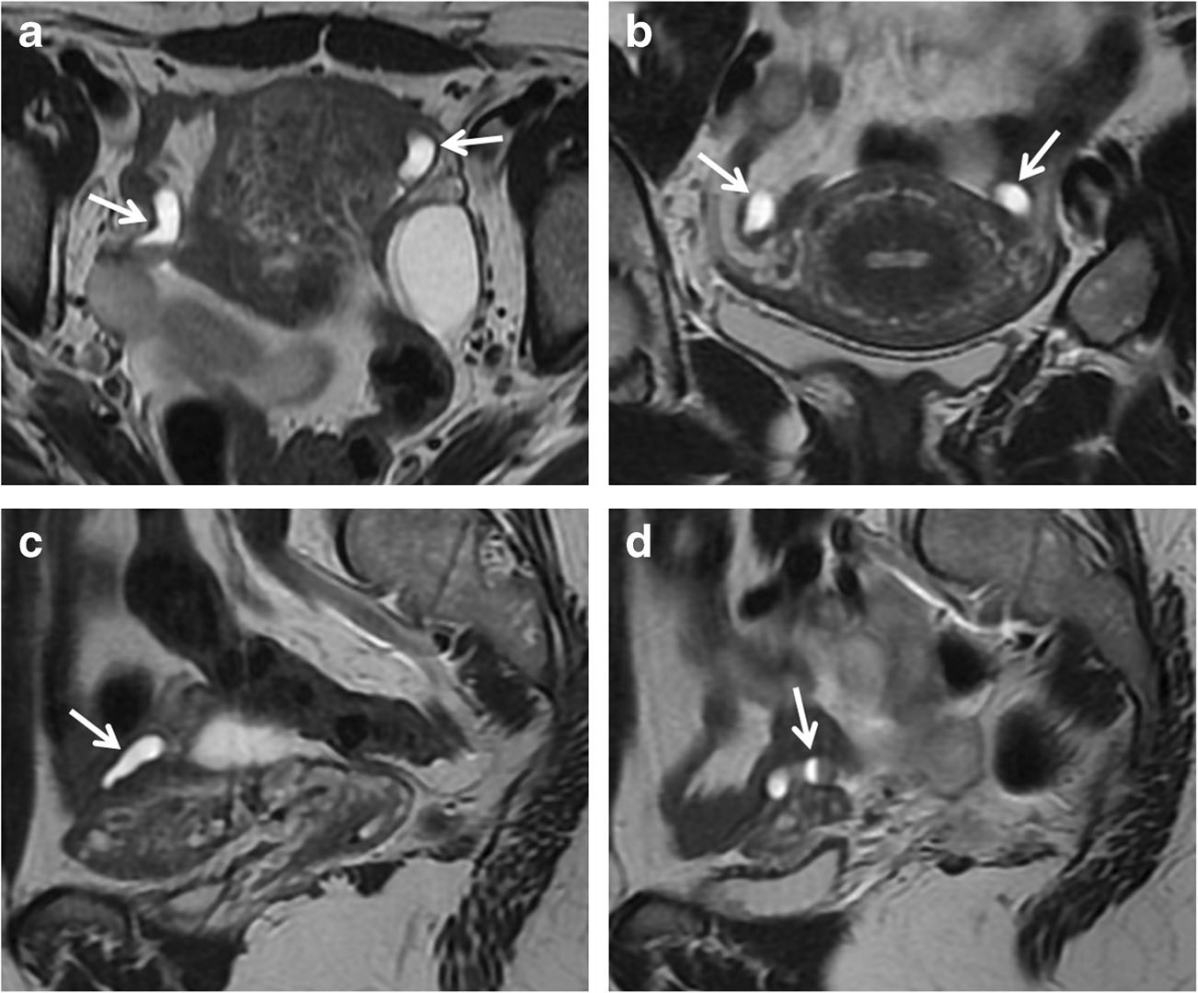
Hydrosalpinx in a 47-year-old woman who underwent tubal ligation. (a) Axial, (b) coronal, and (c, d) sagittal T2-weighted MR images show a paired, serpentine juxtauterine structure located bilaterally at the superior portion of the uterus with hyperintense signal and a fluid-fluid level content (*white arrows*)
